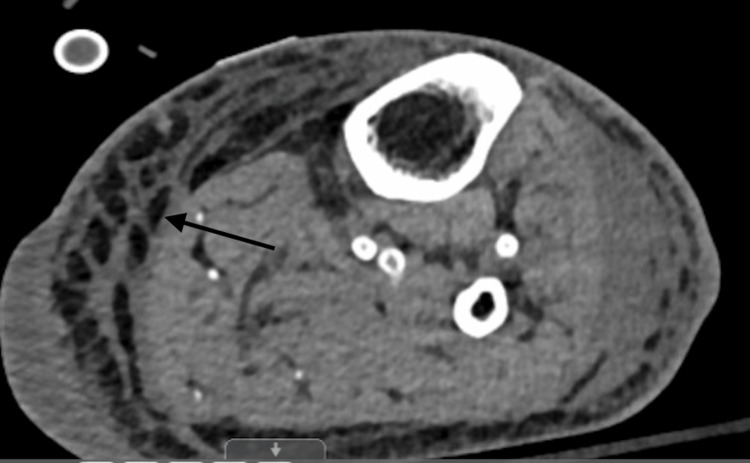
CT tibia and fibula left leg: diffuse soft tissue swelling as evidenced by black arrow
